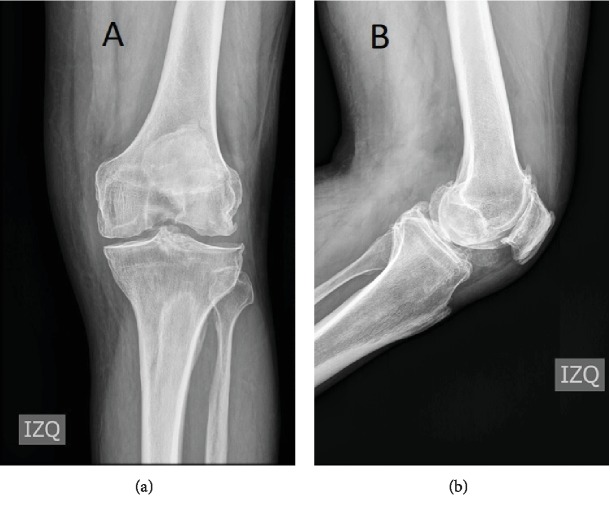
Preoperative radiographs show severe tricompartmental left knee osteoarthritis: (a) anteroposterior left knee view and (b) lateral left knee view.
